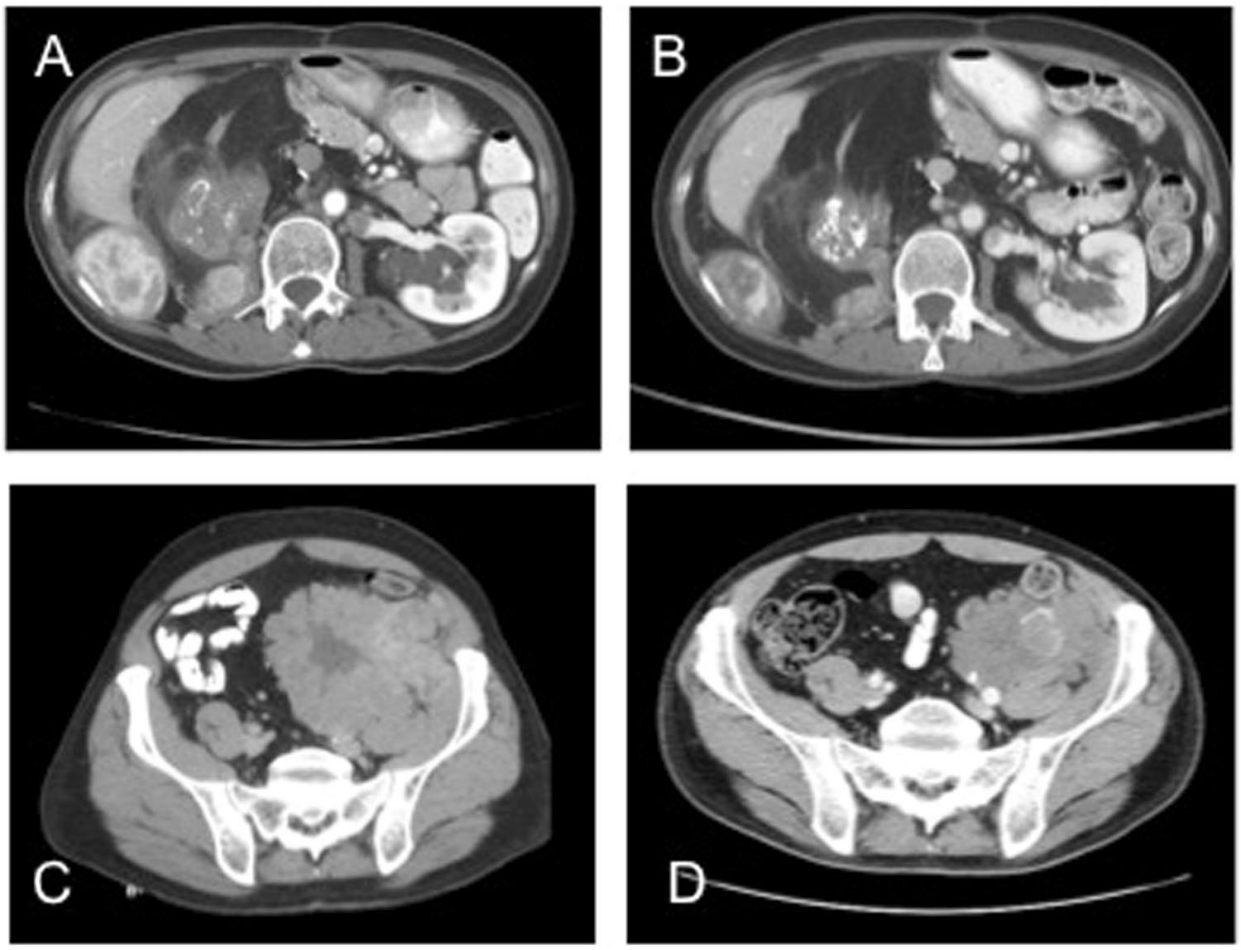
CT Characteristics of Response in WD/DD LPS. (A) Pre- and (B) Post-treatment with response features including decrease vascularity and increased calcification, and increase in fatty elements (WD/ALT); (C) Pre- and (D) Post-treatment with RECIST response and decreased vascularity with increased calcification.
